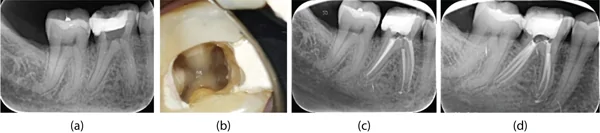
(a) Preoperative radiograph of a mandibular first molar. (b) Photograph of the access cavity showing orifices of three canals in the distal root. (c) Postoperative radiograph with a straight view. (d) Shifted view displaying three independent canals in the distal root.
